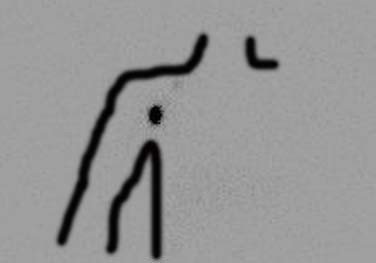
Preoperative lymphoscintigraphy identifies uptake of the radiolabelled tracer in a single axillary lymph node.
